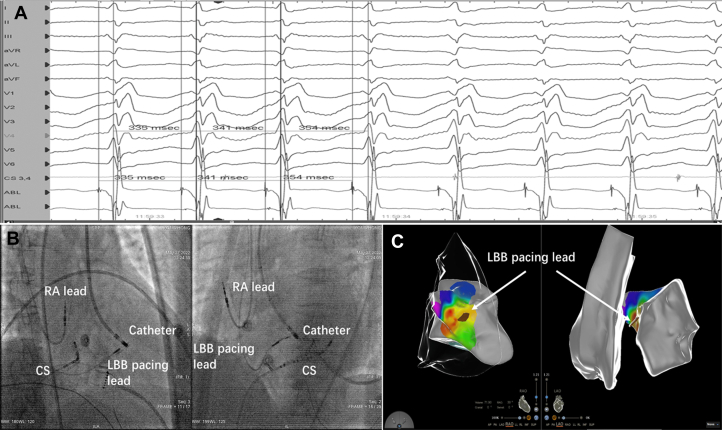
Integrated Electrophysiological and Imaging Findings During VT Mapping and Ablation (A) High-gain intracardiac electrograms during VT showing Purkinje-Purkinje (PP) interval changes preceding RR changes, a diagnostic feature of HPS-related VT. (B) Fluoroscopy in the right anterior oblique and left anterior oblique projections depicting intracardiac catheter positions and the LBBAP lead traversing the interventricular septum. (C) Three-dimensional activation map integrated with intracardiac echocardiography shell shows the earliest LV anterior septal activation immediately adjacent to the LBBP lead marker. CS = coronary sinus; HPS = His-Purkinje system; LBB = left bundle branch; LBBAP = left bundle branch area pacing; LBBP = left bundle branch pacing; LV = left ventricular; RA = right atrial; VT = ventricular tachycardia.
